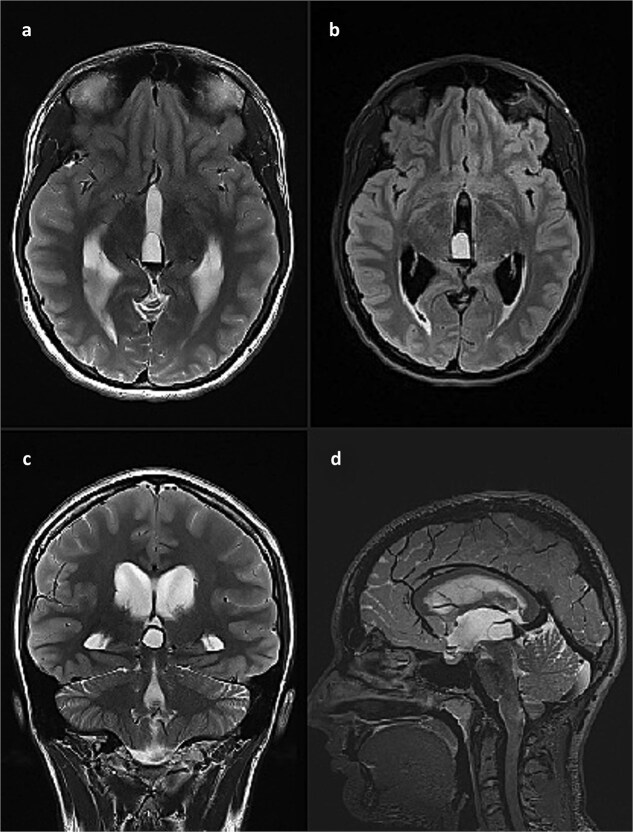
Brain MRI scan. (a) T2 weighted image, axial slice, pineal cyst occupying posterior part of the third ventricle with hemosiderin deposits in the posterior part of the cyst; (b) FLAIR image, axial slice, showing different contents inside the cyst when compared to CSF; (c) T2 weighted image, coronal slice, pineal cyst inside the lower part of the third ventricle, beneath internal cerebral veins; (d) T2 weighted image (SPACE), sagittal slice, showing pineal cyst in front of a rather large vein of Galeni, and compressing the cranial part of the cerebral aqueduct.
